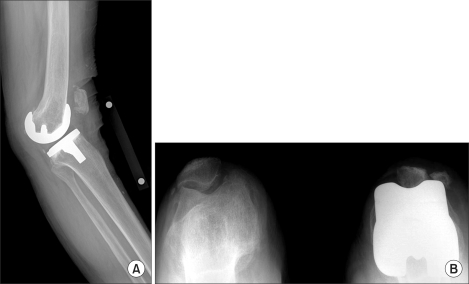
Radiographs taken in the emergency department 14 months after total knee arthroplasty following slipping and falling. (A) Lateral radiograph showing genu recurvatum, (B) 45° Sky line view showing vertical fracture of the left patella.
